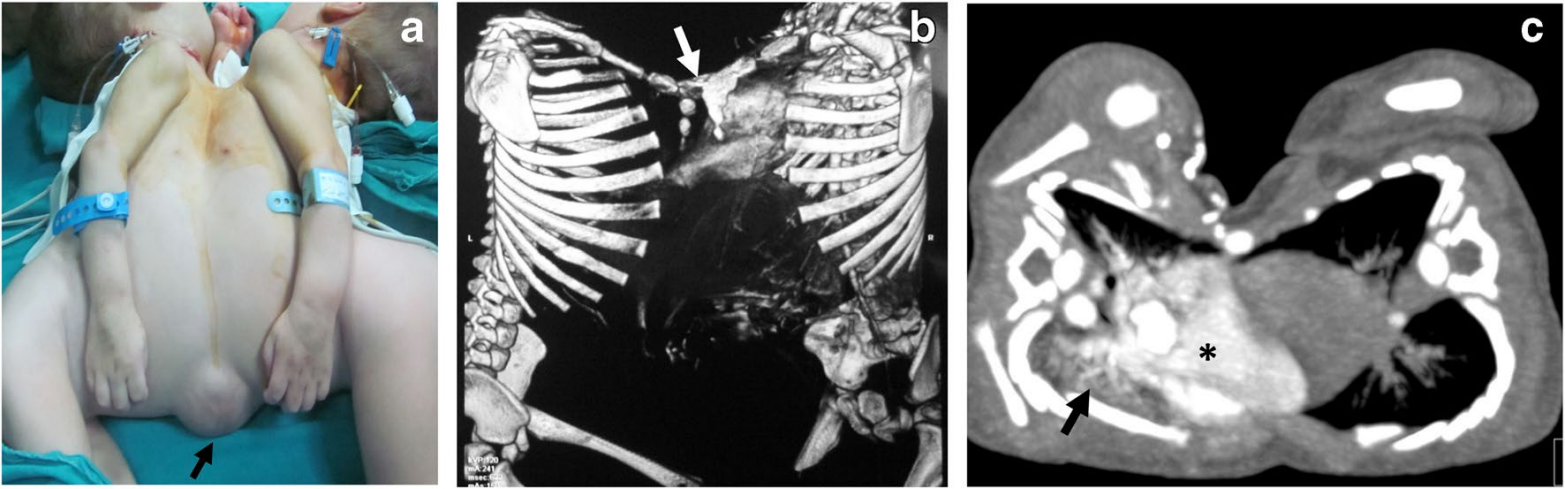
8-month-old thoracopagus conjoined twin boys. a Clinical photographic image shows thoracic and abdominal fusion with a small omphalocele (*arrow*). b 3-dimensional reconstruction of the bony skeleton shows sternal fusion (*arrow*). c Axial computed tomography of the thorax (mediastinal window) following contrast injection into the twin on the right shows opacification of the cardiac chambers of the injected baby only (*asterisk*), denoting separate cardiac chambers. Note the collapsed right lung with increased density (*arrow*)
